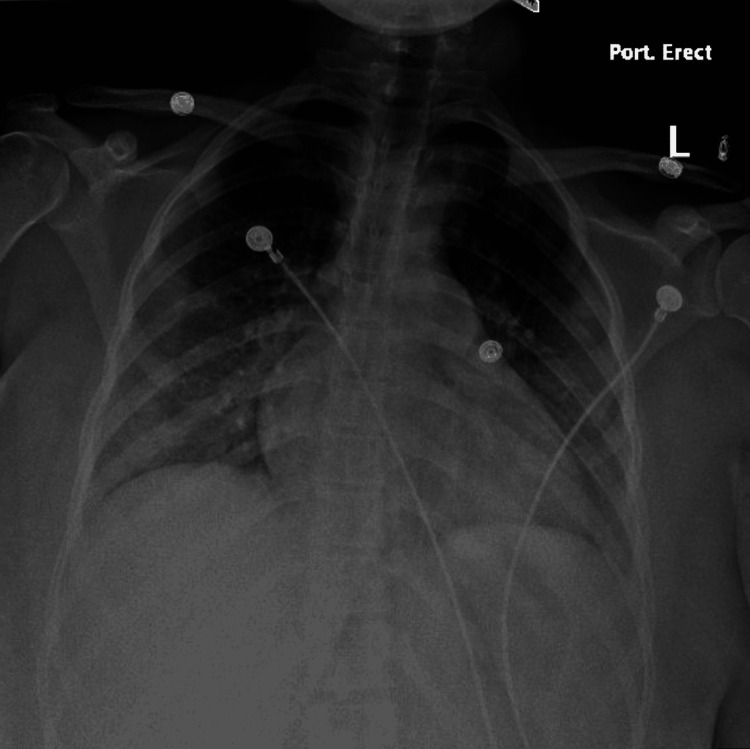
X-ray of the chest showing ill-defined ground-glass opacities in both lungs suspicious for pneumonia/pneumonitis.
